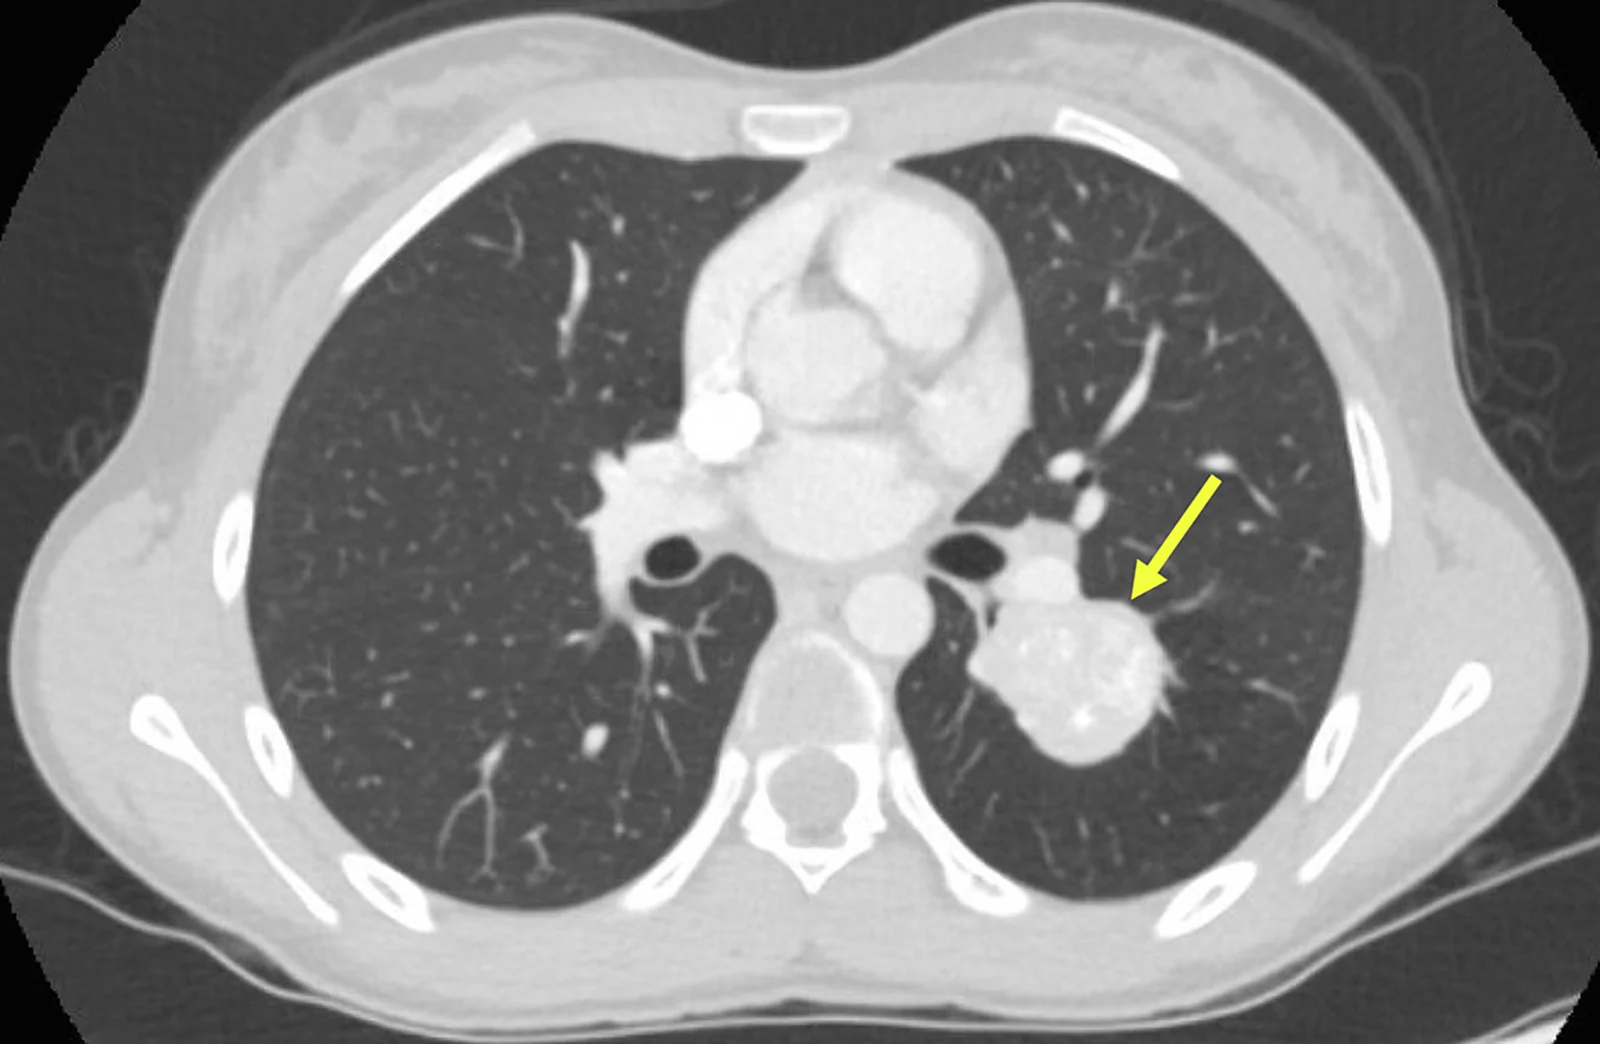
Computed tomography of the chest showed a well-circumscribed mass located in the superior segment of the left lower lobe (arrow).
